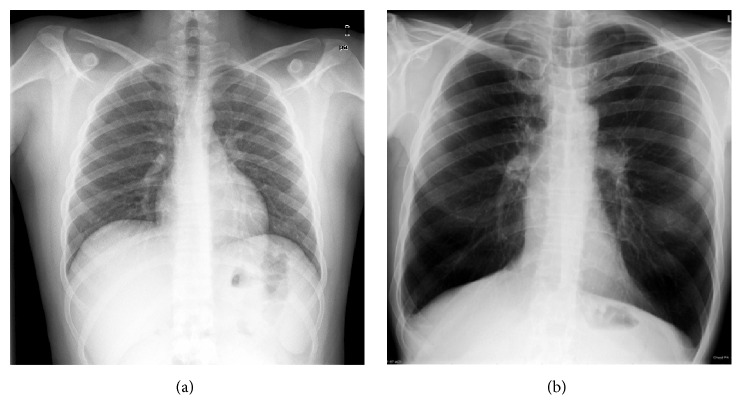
Sample chest radiographs in posteroanterior view. (a) Normal. (b) Pathological.
